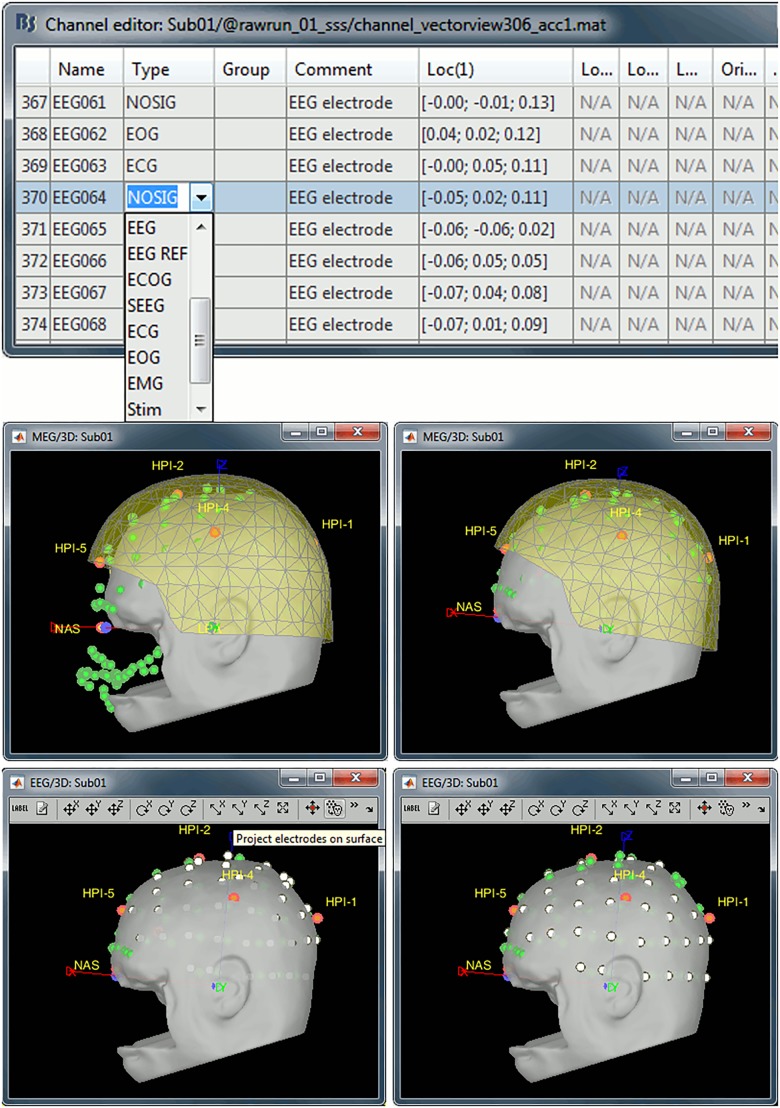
(Top row) Manual editing of channel types, removal of digitized head points below the nasion, and fit of remaining points to the scalp surface; (bottom row) projection of EEG electrodes onto scalp surface. Green dots: digitized head points, white dots: EEG electrodes, gray surface: scalp surface extracted from MRI, yellow surface: inner surface of MEG helmet.
